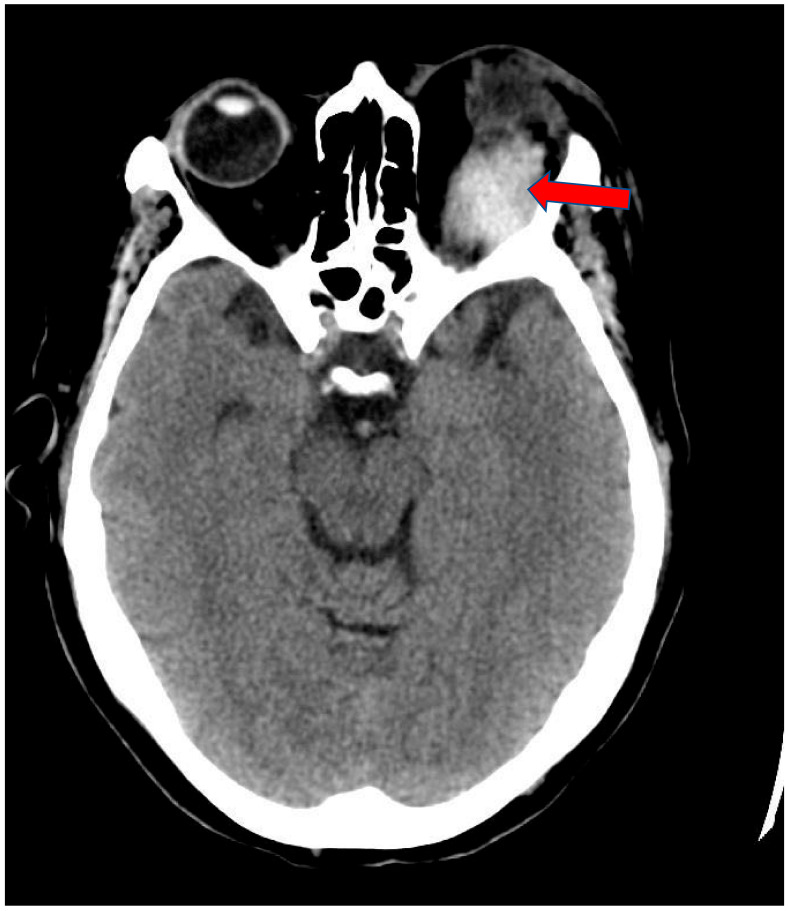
Axial view of Brain CT scan showing left periorbital soft tissue swelling with hyper dense mass. This appears to be located above the superior rectus muscle (Arrow).
